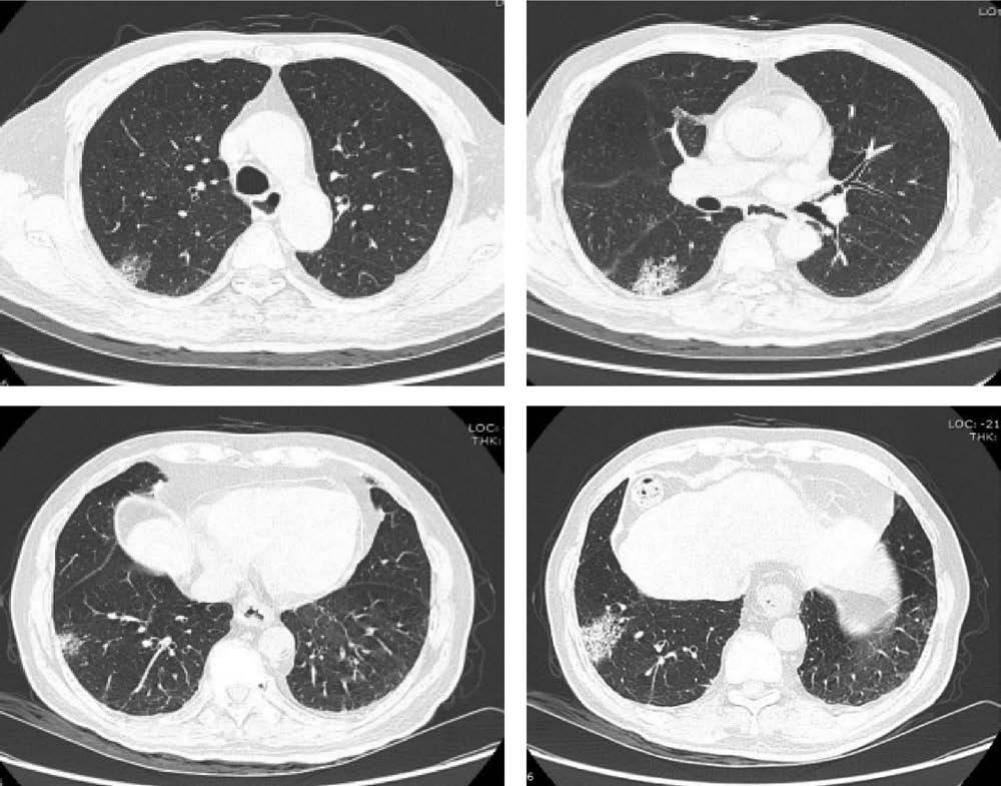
The patient’s COVID-19 antigen and nucleic acid tests turned negative, but a follow-up chest CT scan showed worsening of the lung lesions compared to before.
